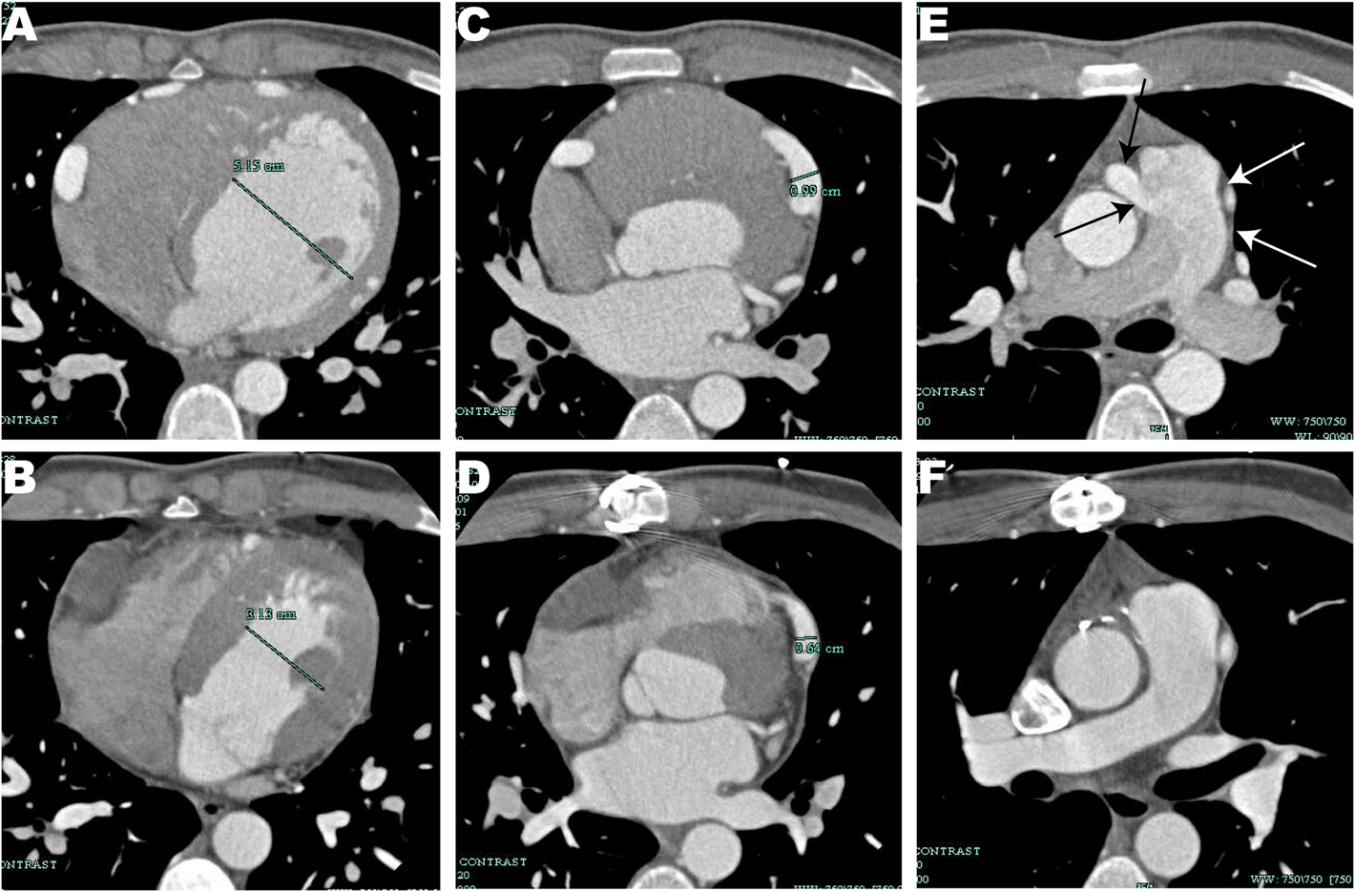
a, c, e Preoperative coronary CT angiography showed RCA arose from the pulmonary artery, causing increased left ventricle size (53 mm), dilated LCA (proximal left anterior descending artery of 10 mm in diameter); b, d, f 5-year postoperative coronary CT angiography showed complete RCA ligation with decreased left ventricle size (31 mm) and less dilated LCA (proximal left anterior descending artery of 6 mm in diameter). CT, computed tomography; RCA, right coronary artery; LCA, left coronary artery
